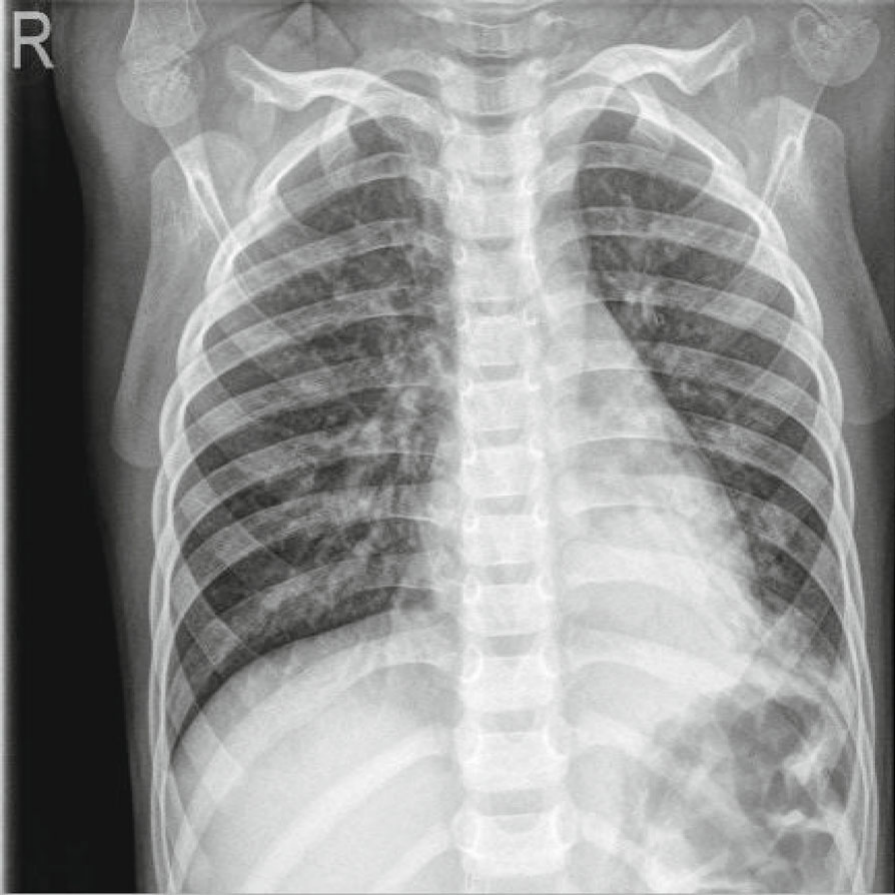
Chest X-ray of patient 1 at admission: atelectasis in the left lower lung
